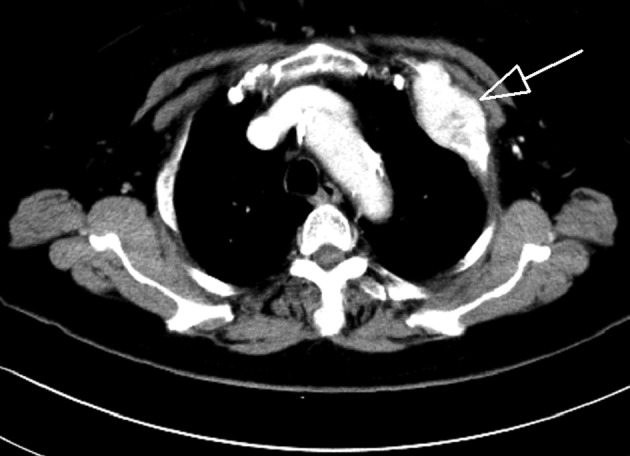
Computed tomography with contrast showing the 2nd rib with bony destruction and a markedly enhanced mass.
